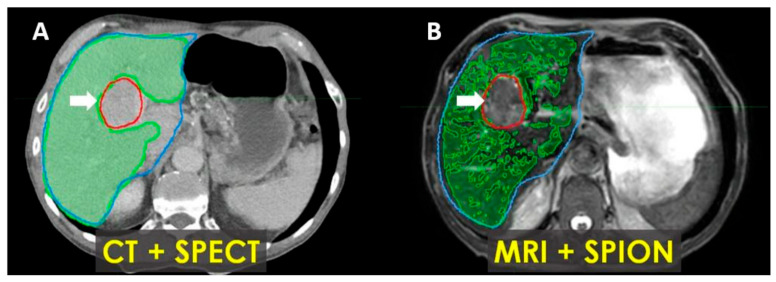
Patient with Child–Pugh A liver cirrhosis: SPECT-CT (A) and MRI-SPION (B) imaging of tumor (arrow) and functional liver volumes (FLVs) (green). Anatomic liver volume delineated in blue. There is an estimated 20% loss of functional hepatic parenchyma, with more accurate tumor delineation defined via MRI-SPION compared to SPECT/CT.
